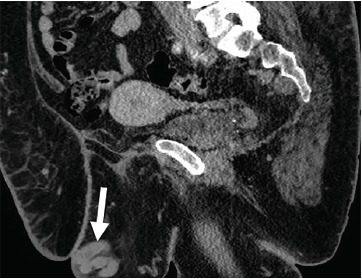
Sagittal cross-section of computed tomography of abdomen and pelvis with intravenous contrast demonstrating dilated pelvic veins (arrow) near the mons pubis.
